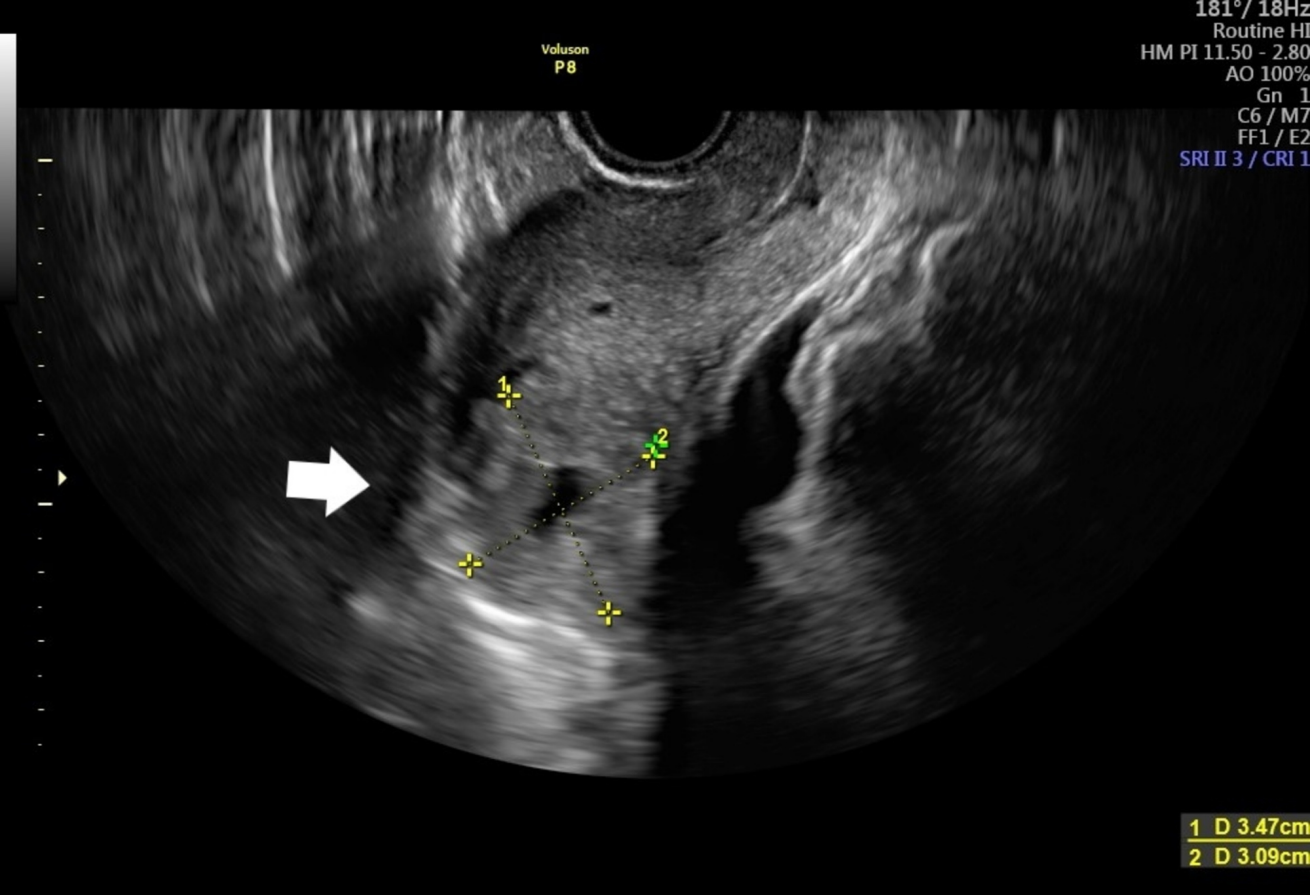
Transvaginal ultrasound Transvaginal ultrasound indicating echogenic structure in left cornual region (arrow).
